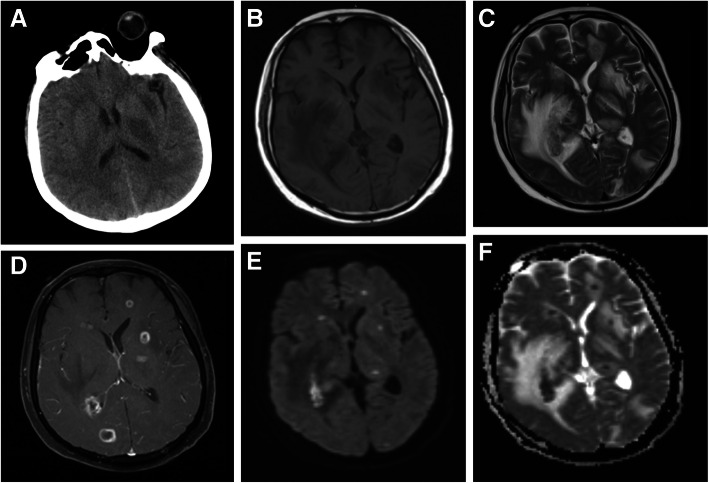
Imaging changes of the patient’s brain. CT (a) and MRI (b-f) indicates brain abscess
